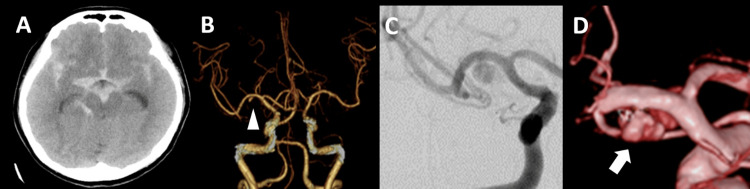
Findings on admission (A) CT upon admission showing diffuse subarachnoid hemorrhage. (B) CT angiography showed a small aneurysm in the middle cerebral artery M1 (arrowhead). (C) DSA showing delayed contrast and congestion findings suspicious for a pseudoaneurysm. (D) Three-dimensional DSA showing a pseudoaneurysm contiguous with a saccular aneurysm at the non-branching segment in the middle cerebral artery M1 (white arrow) CT: computed tomography; DSA: digital subtraction angiography; M1: middle cerebral artery M1 segment
